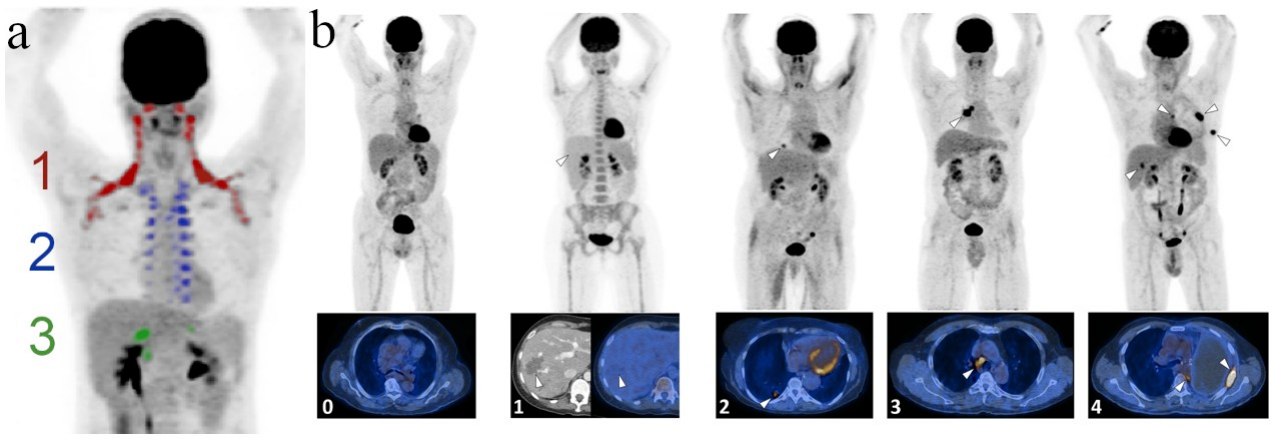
2a: Coronal FDG-PET projection showing the anatomical locations of the BAT depots (color coded: 1 = supraclavicular/cervical, 2 = thoracic, 3 = infradiaphragmatic/abdominal activation). BAT in humans is strictly activated in a craniocaudal fashion, i.e. the more caudally activated depots indicate higher overall glycolysis and can hence be categorically graded in this way. 2b: Representative examples of patients suffering from different grades of cancer burden. Grades were assigned as follows: 0 = No visible signs of cancer, 1 = cancer visible in CT, but metabolically inactive in FDG-PET, 2 = single lesion, 3 = local metastases, 4 = distant metastases. Top row: Maximum intensity projections of the FDG-PET data (dark = high FDG uptake), bottom row: fused PET/CT slices through representative locations (yellow = high FDG-uptake). Index lesions are marked with white arrow tips.
